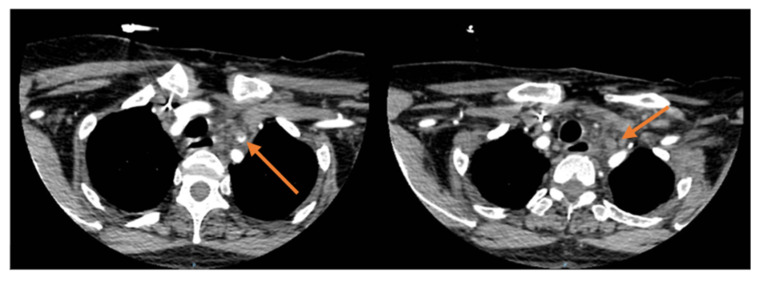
Postoperative CT scan showing thrombosis of the carotid bypass graft.
